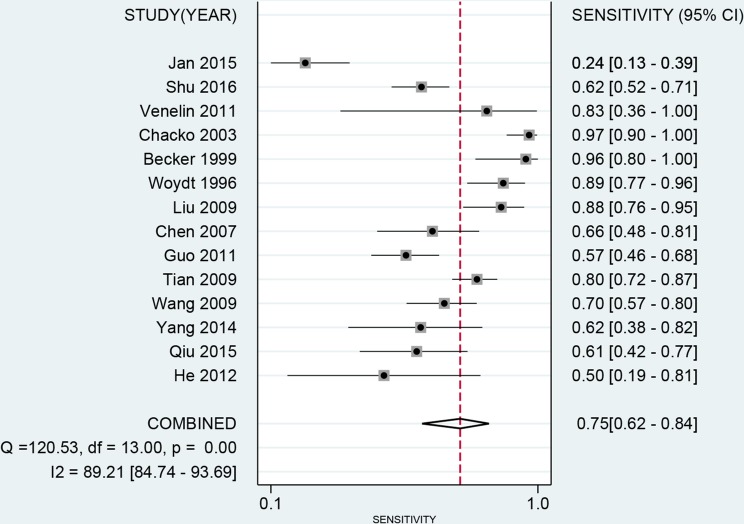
Forest plot of pooled sensitivity of intraoperative ultrasound for glioma residual
